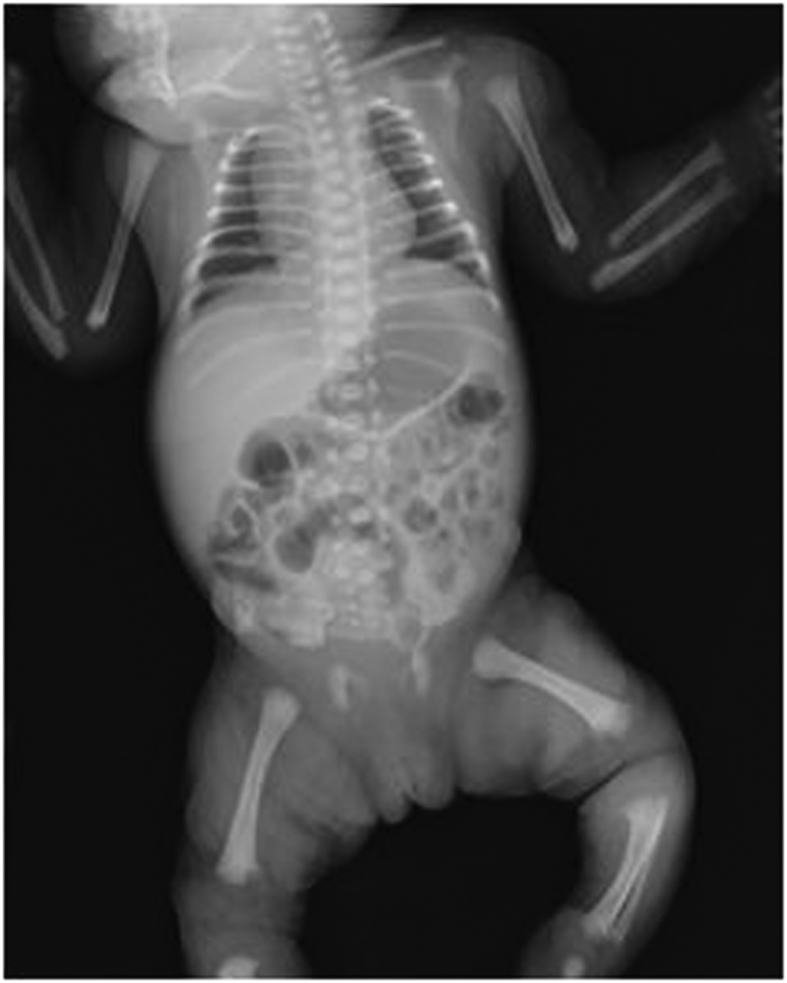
Radiograph of patient at birth. It showed typical findings such as significant platyspondyly, shortening of long bones with ragged metaphyses, mild iliac hypoplasia, and delayed ossification of femur head with absence of pubic ossification delay.
